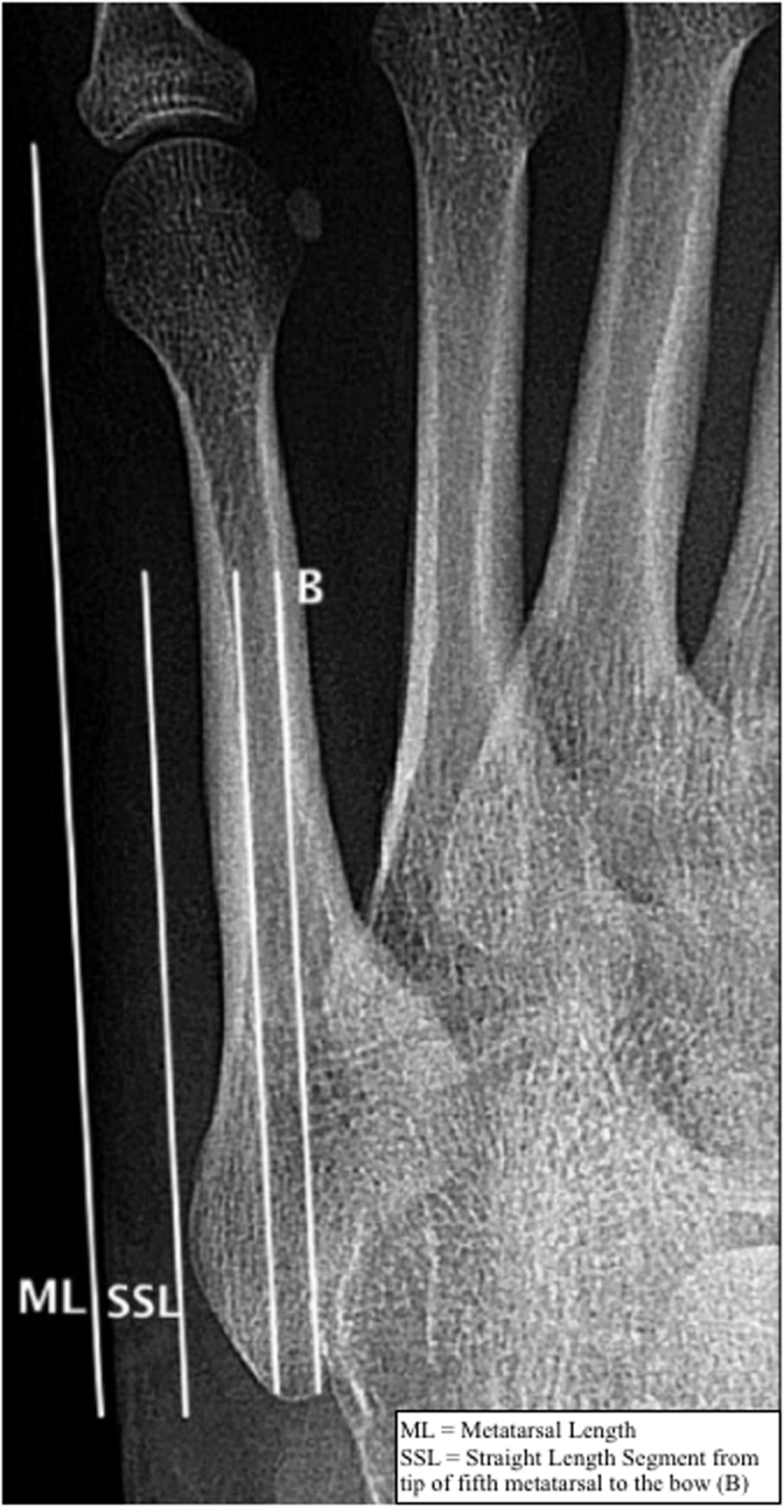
Radiographic measurements pertaining to the fifth metatarsal. AP radiograph demonstrating how measurements pertaining to the fifth metatarsal were performed. The medullary canal diameter was measured at the bow of the metatarsal (labeled B)
